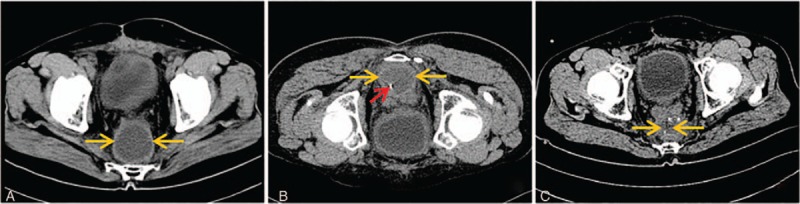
Presacral space approach. (A) CT scans revealed that 5 × 7 cm abscess (light orange arrow) is located between bladder and sacrum (the rectum had been resected). (B) When the patient was in prone position, intraprocedural CT scans demonstrated that the tip of needle (red arrow) advanced into presacral abscess (light orange arrow) during procedure. (C) CT scans obtained 3 days after the procedure revealed that abscess cavity (light orange arrow) obviously shrinked.
